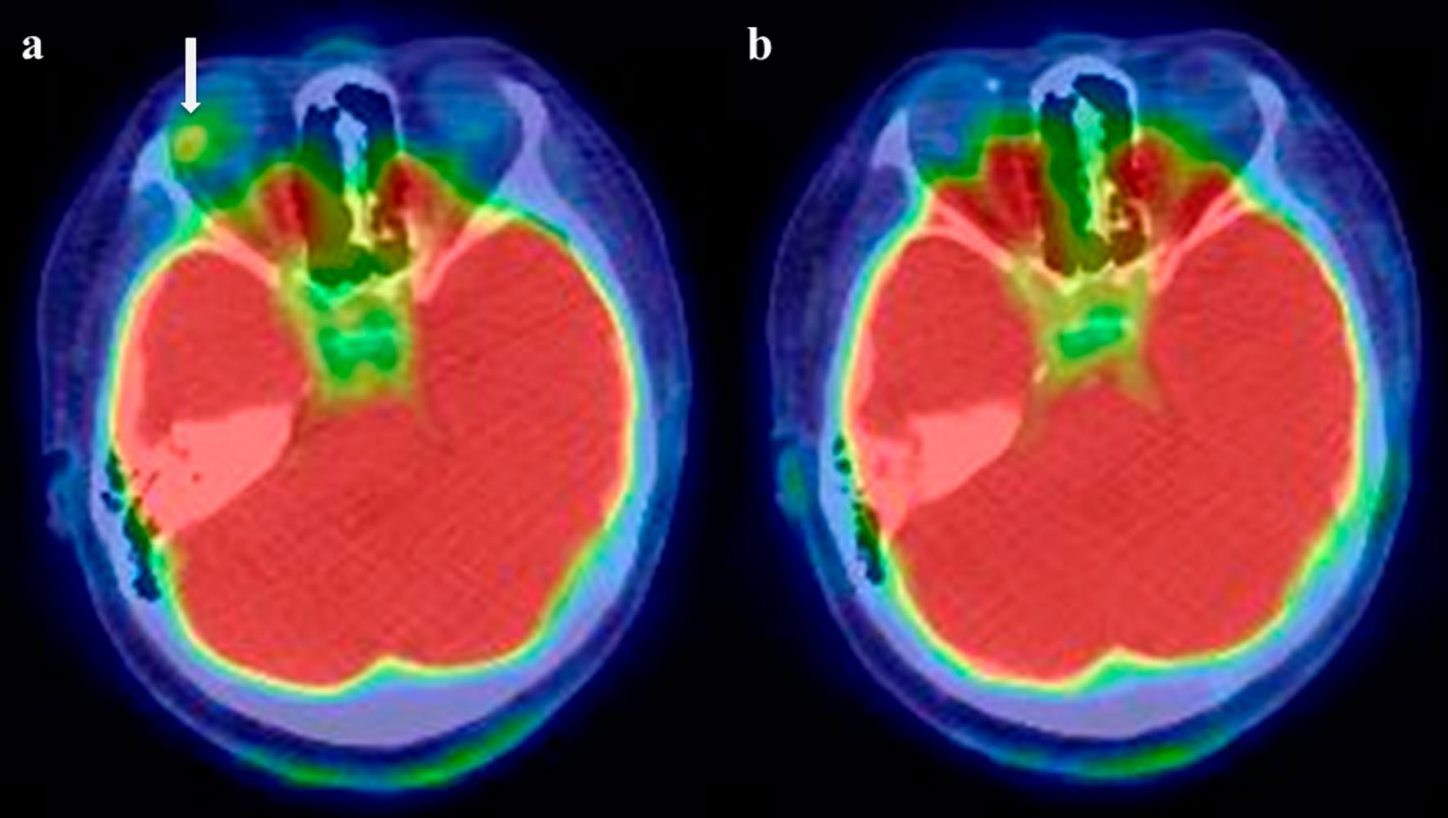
Pre-treatment positron emission tomography (PET) showed significant fluorodeoxyglucose (FDG) accumulation in the right eye (a). PET after heavy-particle radiotherapy showed no FDG accumulation in the lesion (b)
